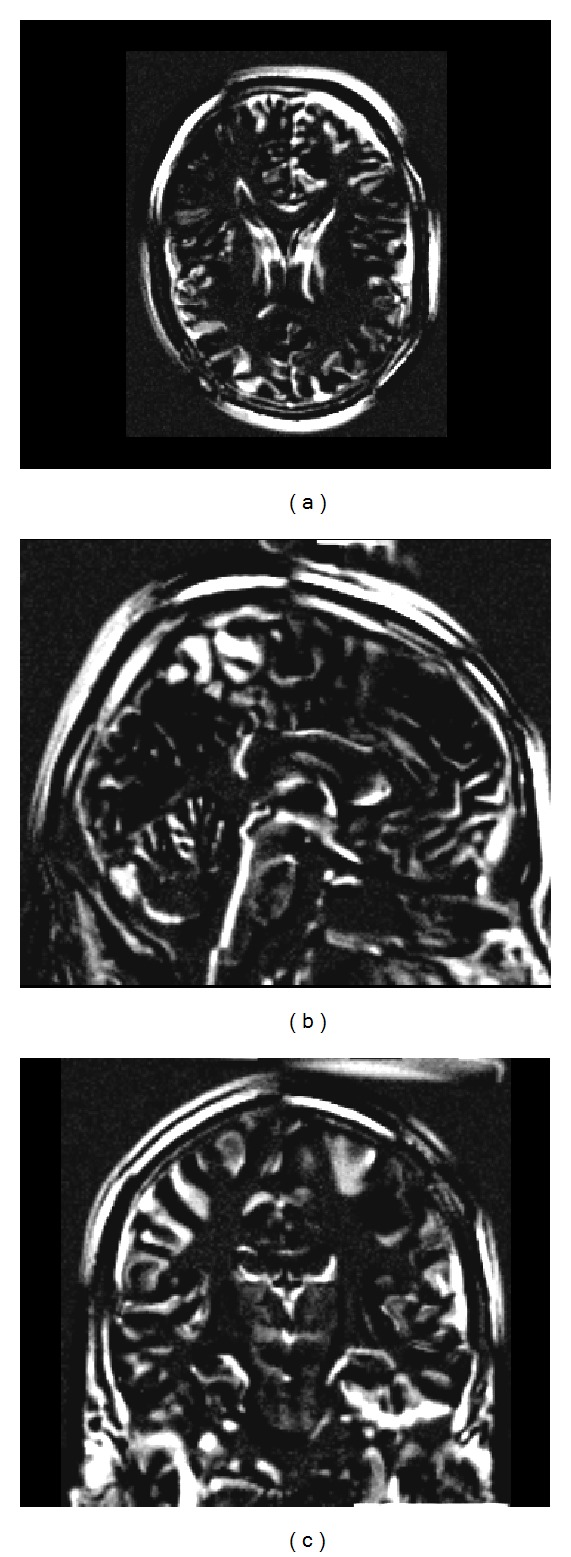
Image difference between the original T1-weighted BrainWeb volume and one of the supplemental volumes generated through a thin plate spline-based three-dimensional deformation grid. (a) Axial (b) sagittal, and (c) coronal views.
